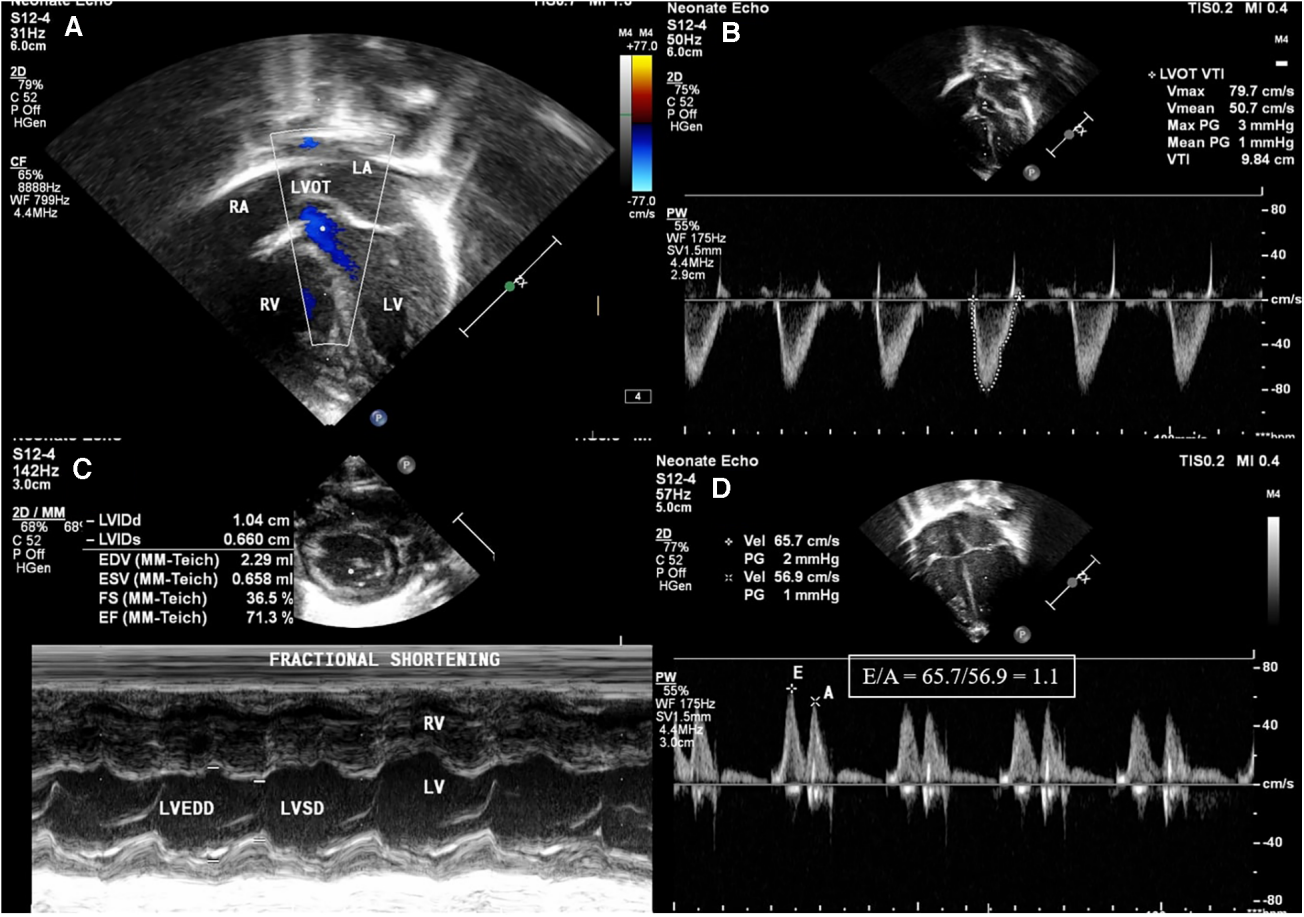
Images of left ventricular systolic and diastolic function parameters in echocardiography. (A) Left ventricular outflow tract (LVOT) seen in apical 5 chamber view. (B) Left ventricular velocity time integral measured in apical view with LVOT using pulse wave Doppler (VTI): shown in the figure as 9.84 cm. (C) Fractional shortening (FS) in the parasternal short axis (PSAX) view using M-mode using equation FS = LVEDD-LVESD/LVEDD. (D) Apical 4 chamber view pulse wave Doppler across mitral valve showing E/A ratio of 1.1.
